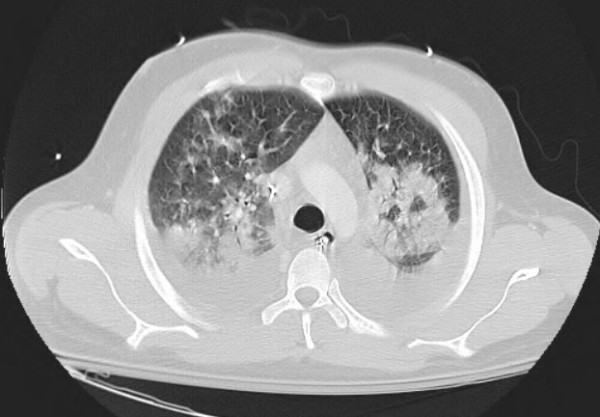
Computed tomography of the chest showed diffuse alveolar infiltration bilaterally with pleural effusions.
